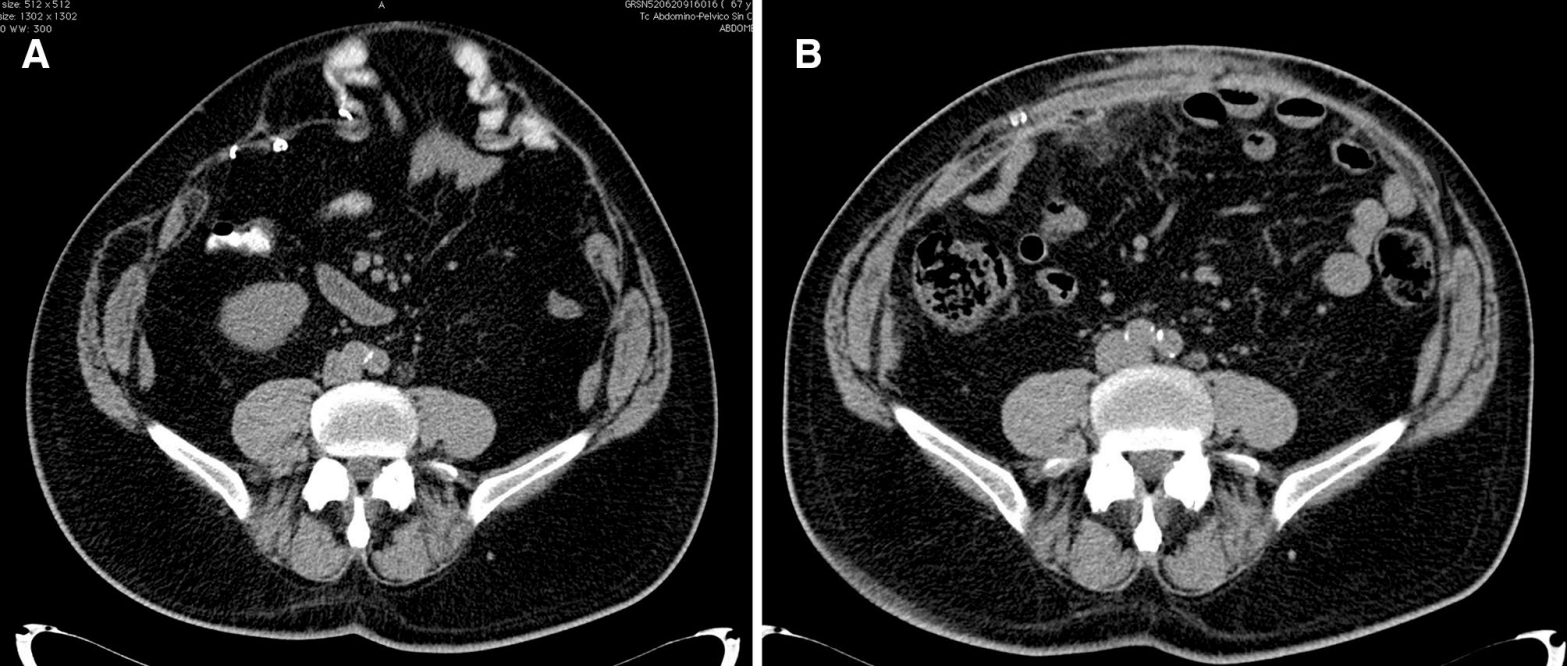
a Preoperative CT scan of a 58 years old man with a midline defect 28 cm long and maximum 22 cm width. b Control CT scan without Valsalva at 4 postoperative months of the patient a after AWR with the combination of TAR and external oblique release
